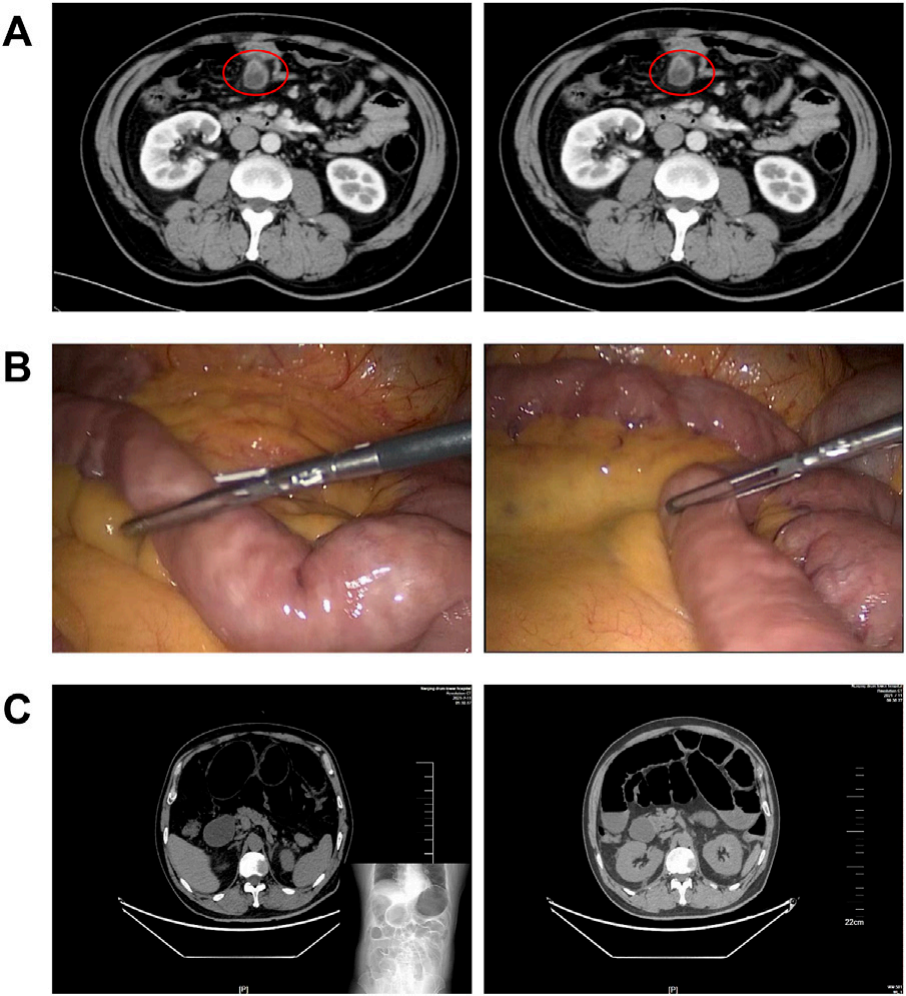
Gut manipulation in the abdominal surgery leads to postoperative ileus. (A) CT imaging features of a patient with small bowel diverticulum (red circles); (B) photos of intestinal manipulation during endoscopic surgery of this patient; and (C) postoperative imaging suggested that this patient had severe ileus.
